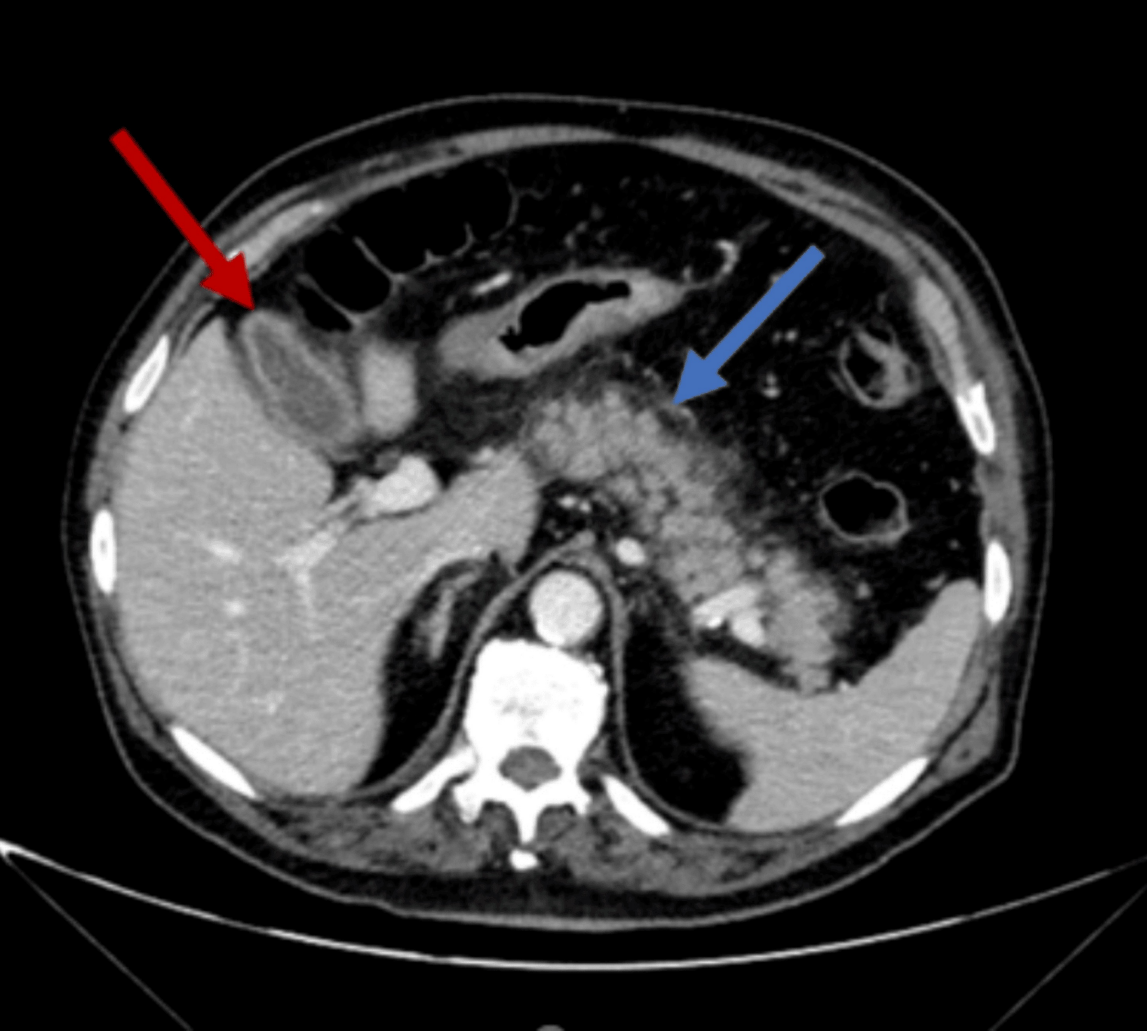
Abdominal CT scan (axial) reveals a slightly thickened gallbladder wall with pericholecystic fat stranding (red arrow), suggestive of cholecystitis. Additionally, the pancreas appears enlarged with peripancreatic fat stranding (blue arrow). No fluid collections or free fluid are seen within the peripancreatic space.
